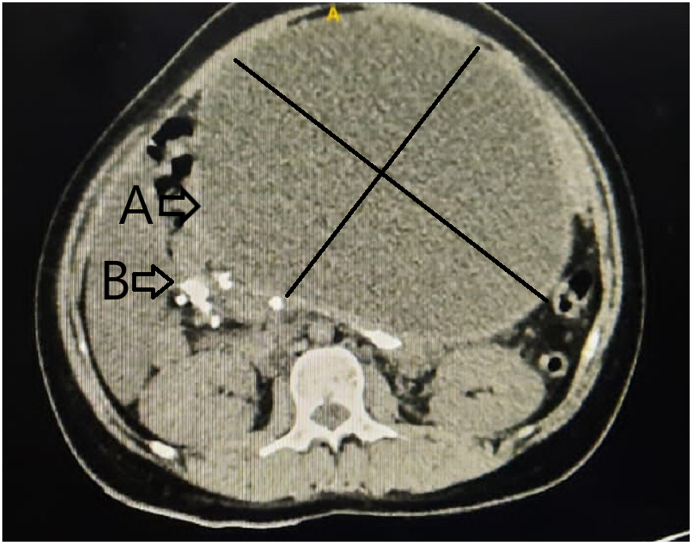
Abdominal CT scan demonstrated: (A) Large pseudocyst pancreas (B) Multiple stones in the major pancreatic duct and the largest stone in the pancreatic head.
